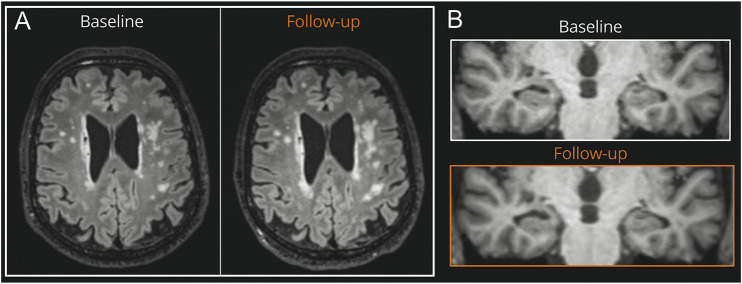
Example of WMHV Change and Hippocampal Atrophy An example of widespread WMH growth on co-registered axial FLAIR images (A) and hippocampal atrophy on coronal T1 images of the temporal lobes (B) at baseline and follow-up 2.5 years later. In this individual, the WMHVR was 4.6 mL/y, the baseline total hippocampal volume was 6.6 mL, and the HAR was 0.17 mL/y. FLAIR = fluid-attenuated inversion recovery; WMH = white matter hyperintensity; WMHV = WMH volume; WMHVR = WMHV change rate.
